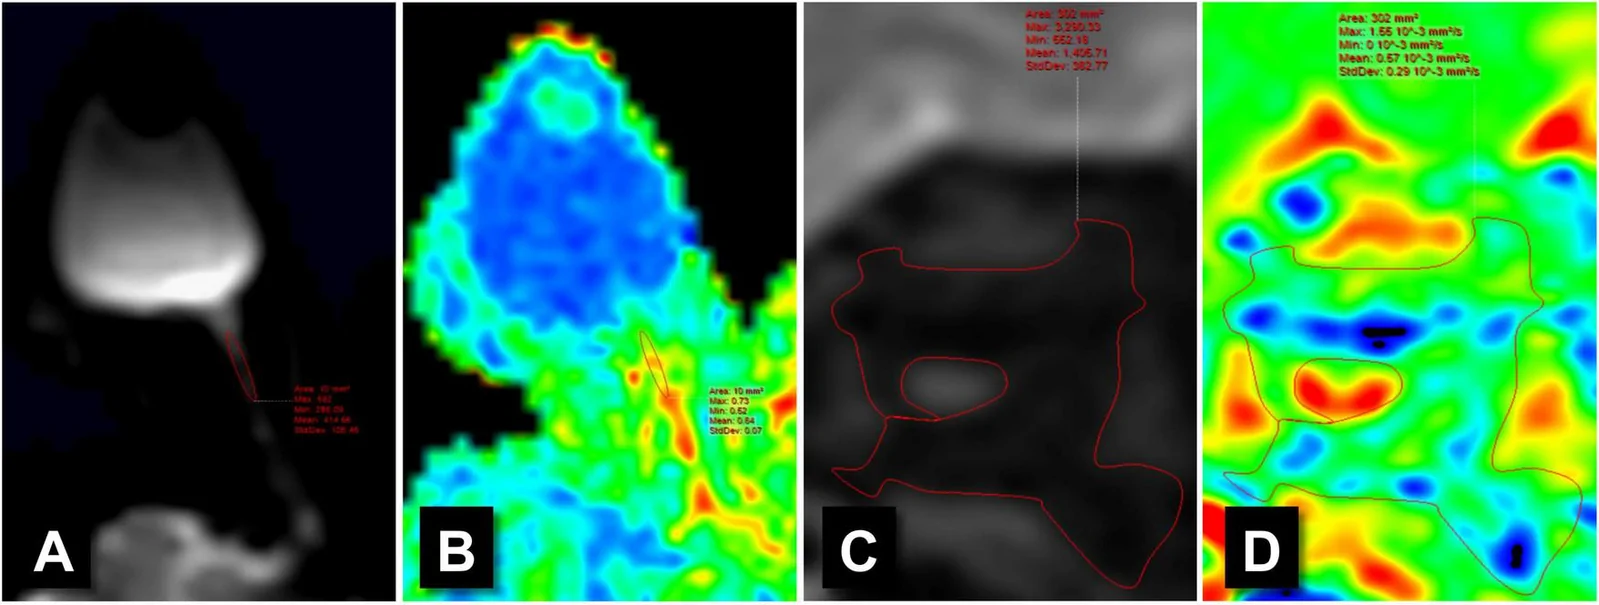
Measurement of orbital diffusion-related MRI parameters. (A,B) A 10 mm^2^ ROI was placed on the optic nerve on axial DTI images (A) and mapped to color-coded FA maps (B) for FA quantification; (C,D) ROIs of the intraconal fat space were drawn on DWI images (C), and ADC values were obtained on corresponding ADC maps (D). ADC values of the optic nerve and extraocular muscles were measured similarly. ROI, region of interest; DTI, diffusion tensor imaging; FA, fractional anisotropy; DWI, diffusion-weighted imaging; ADC, apparent diffusion coefficient.
